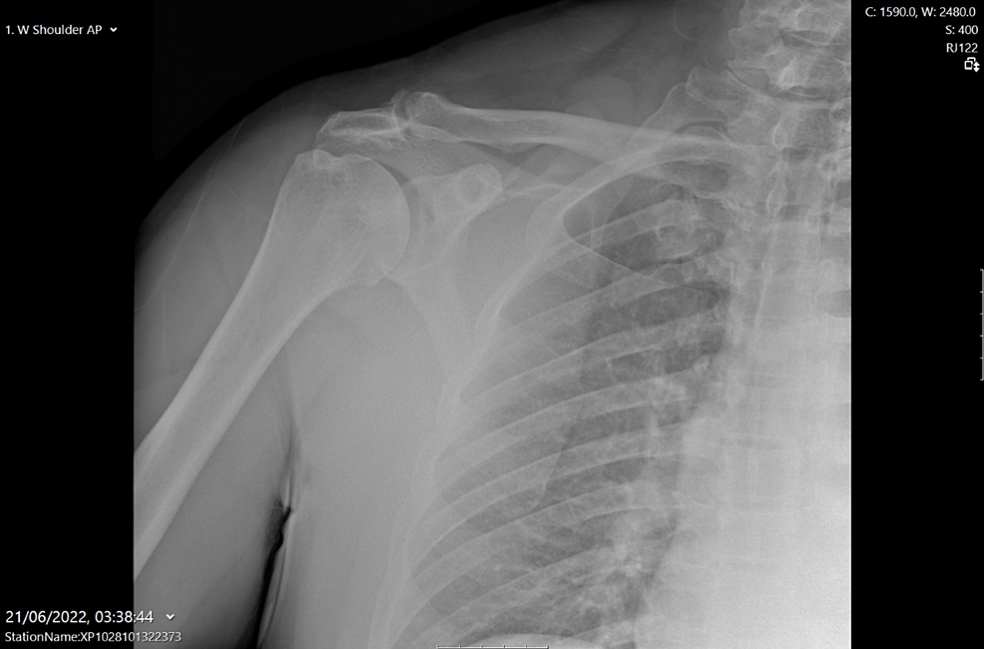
Right shoulder x-ray No bony abnormalities are evident
